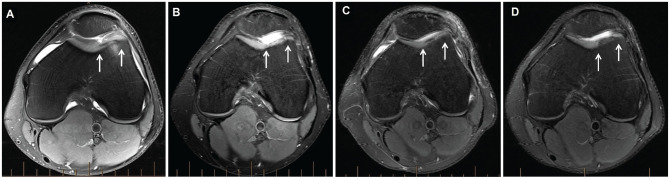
Axial proton density–weighted fat-saturated magnetic resonance imaging of a graft (between white arrows) on the trochlea in a patient, demonstrating (A) the preoperative chondral defect and the graft at (B) 2 years, (C) 5 years, and (D) final follow-up (minimum, 10 years after surgery).
